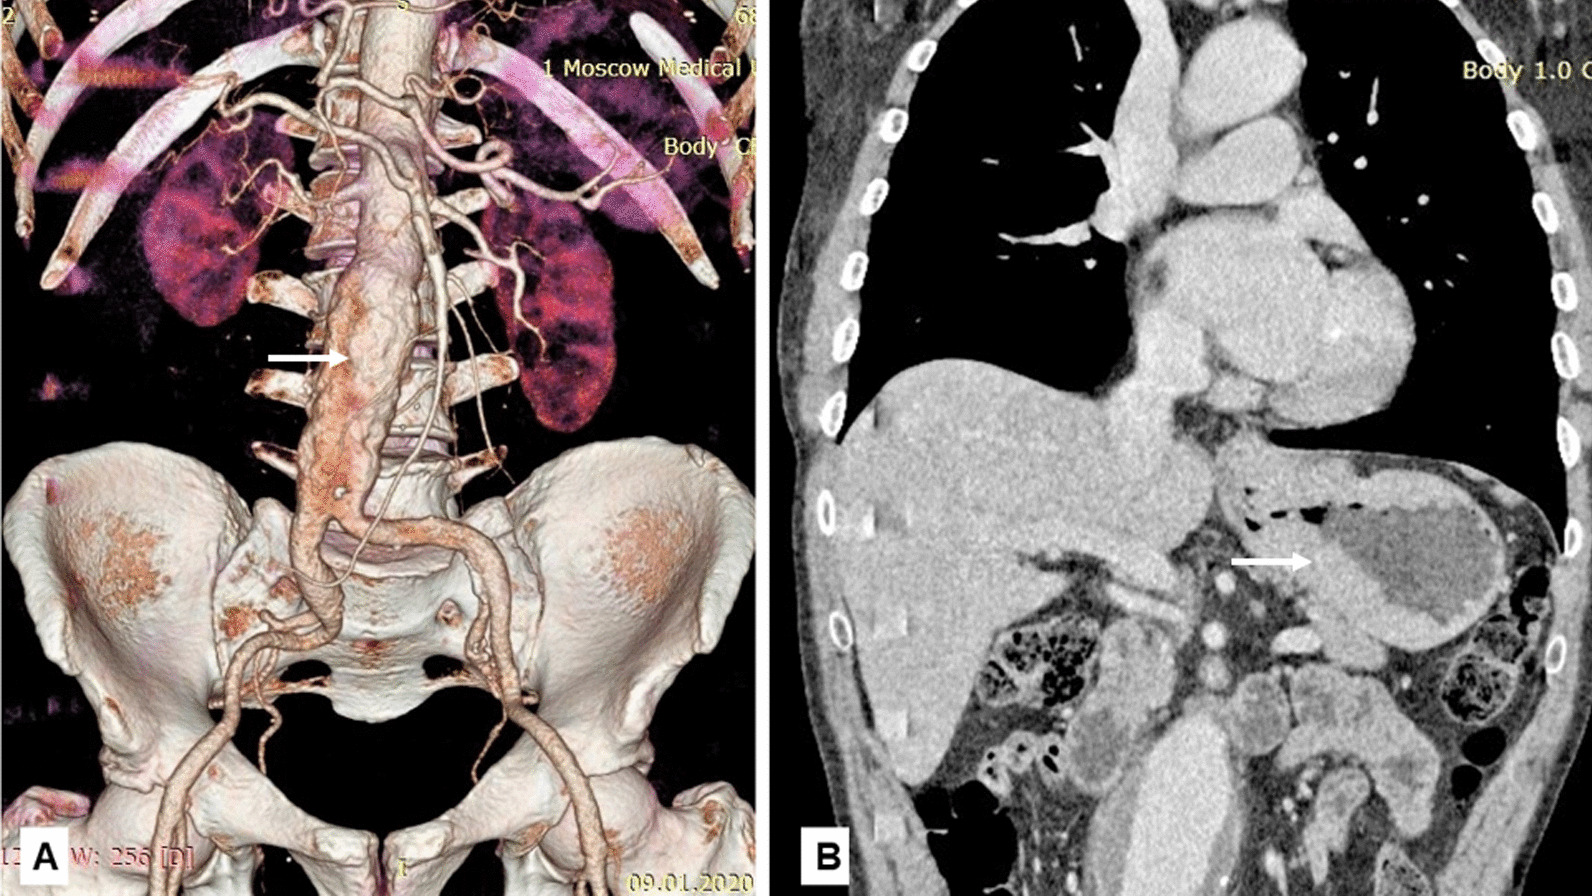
Computed tomography scans of the patient before surgery: A 3D reconstruction (the arrow indicates abdominal aortic aneurysm); B computed tomography scan of the chest and abdomen (the arrow indicates the lesion of the stomach)
